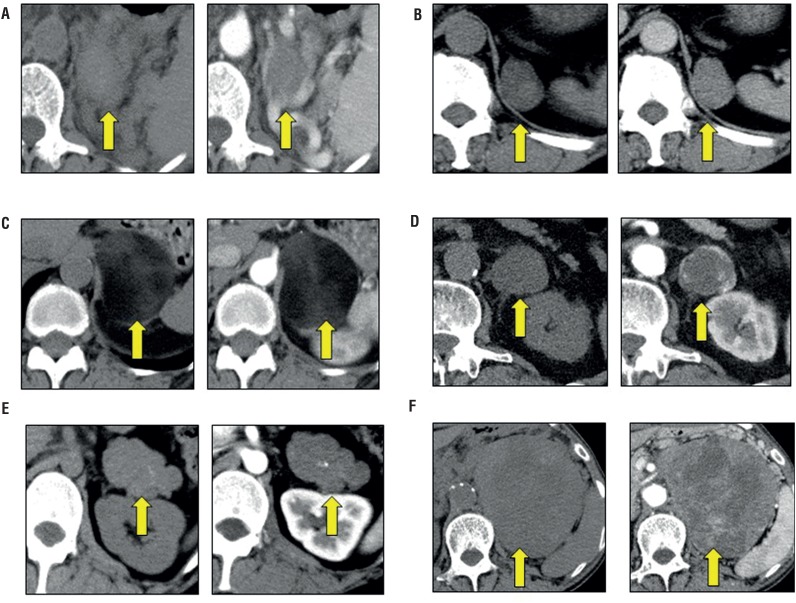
CT images of surgically resected AIs. (a) Adrenal hematoma. (b) Pseudocyst. (c) Myelolipoma. (d) Adrenal hemangioma. (e) Ganglioneuroma. (f) Adrenal carcinoma. Arrows indicate the adrenal tumors.
